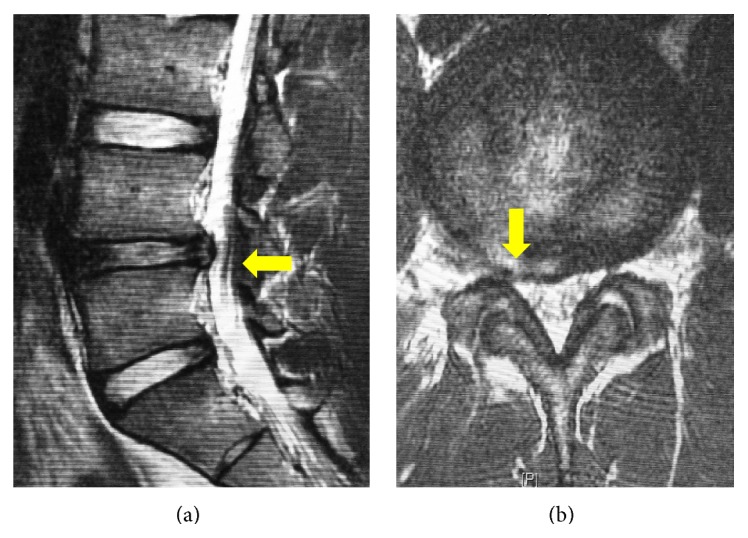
Postoperative magnetic resonance imaging at the 2-month follow-up: (a) sagittal T2-weighted and (b) axial T2-weighted images demonstrating complete resolution of the cystic lesion (arrows).
